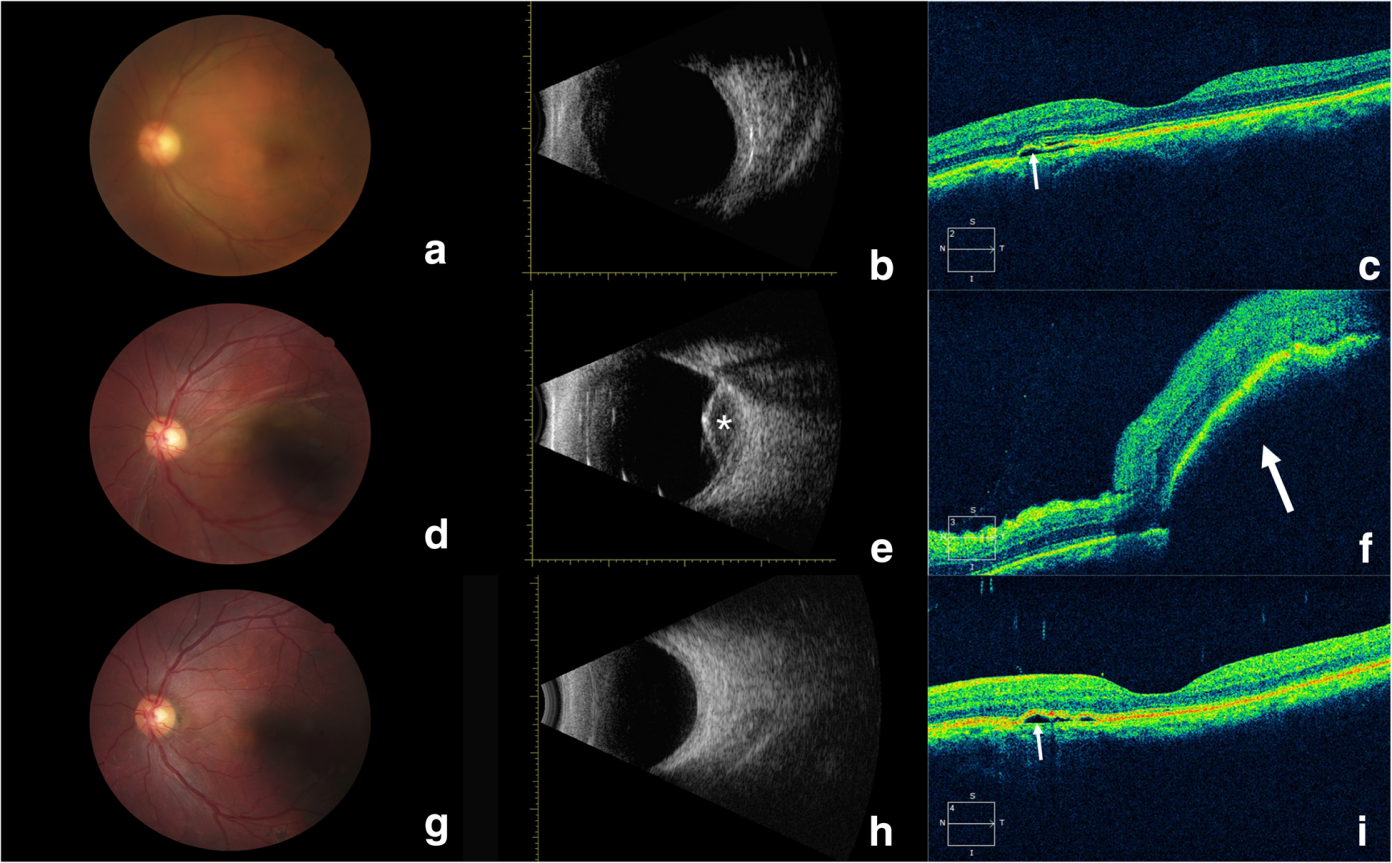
Changes in the eye with uveal effusion. a, b Preoperative fundus photography (a) and type B ultrasonography (b) were unremarkable. c Preoperative OCT revealed fluid accumulation (*arrow*) beneath the neurosensory retina, resulting in mild serous detachment. d Fundus photography on postoperative day 1 revealed suprachoroidal exudation in the macula. e Type B ultrasonography on postoperative day 1 revealed serous choroidal detachment, with little blood at the posterior pole (asterisk). f OCT on postoperative day 1 revealed a bulging macular choroid with edematous neuroepithelium and subfoveal effusion (*arrow*). (g, h) Fundus photography (g) and type B ultrasonography (h) on postoperative day 50 were unremarkable. i OCT on postoperative day 50 revealed complete resolution of the uveal effusion and mild serous detachment of CSC (*arrow*)
